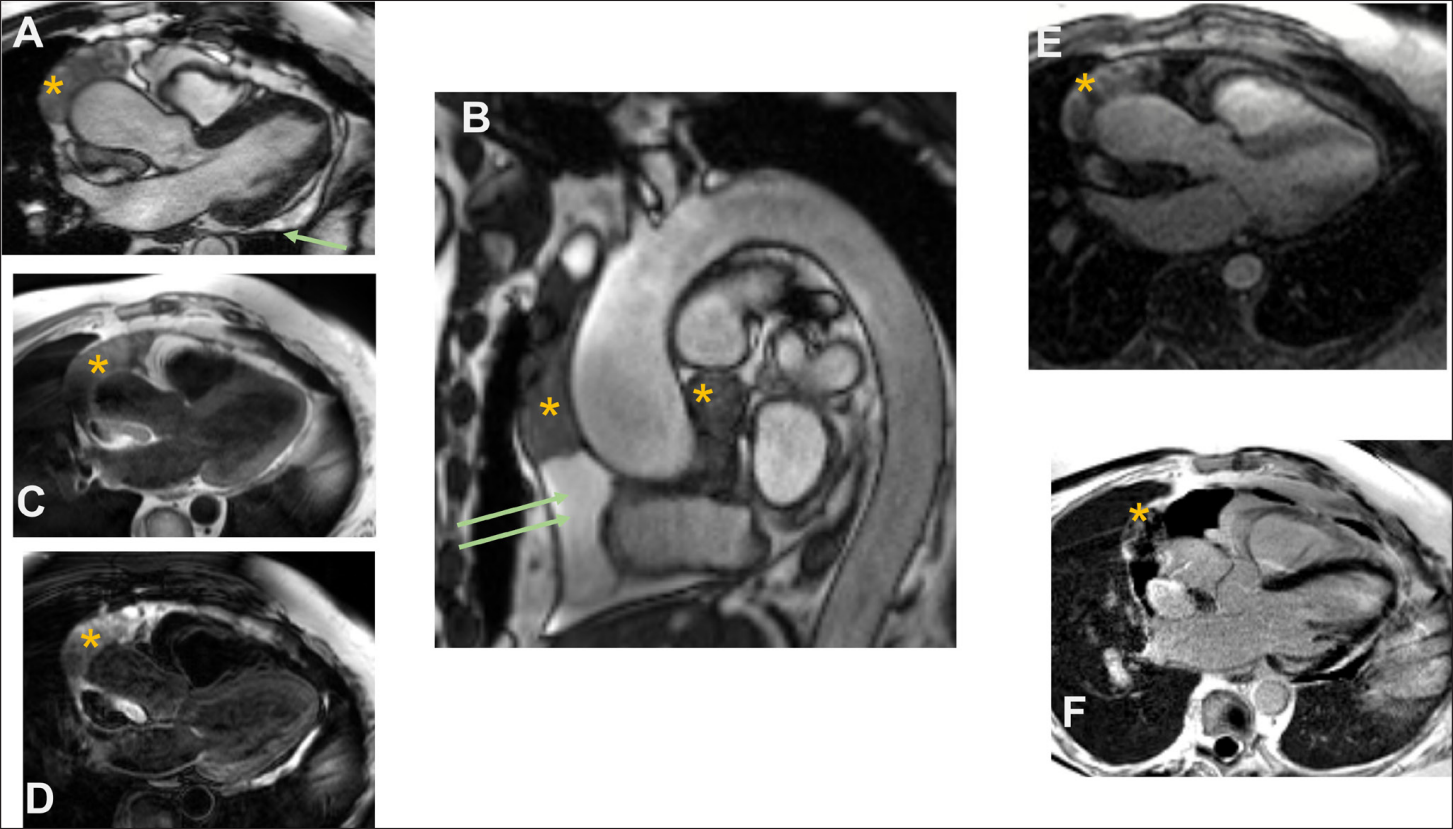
Cine cardiac magnetic resonance imaging. (A) 3-chamber view showing heterogenous lobulated intrapericardial mass (asterisk) with a posterior pericardial effusion (arrow). (B) Sagittal candy-cane view of the aorta showing pericardial effusion (arrows) and masses (asterisk). (C-D) T1- and T2-weighted imaging showing isointensity on T1 and hyperintensity on T2. (E-F) First pass perfusion and late gadolinium imaging showing evidence of contrast uptake on the first pass and heterogenous signal intensity suggesting patchy late gadolinium uptake.
